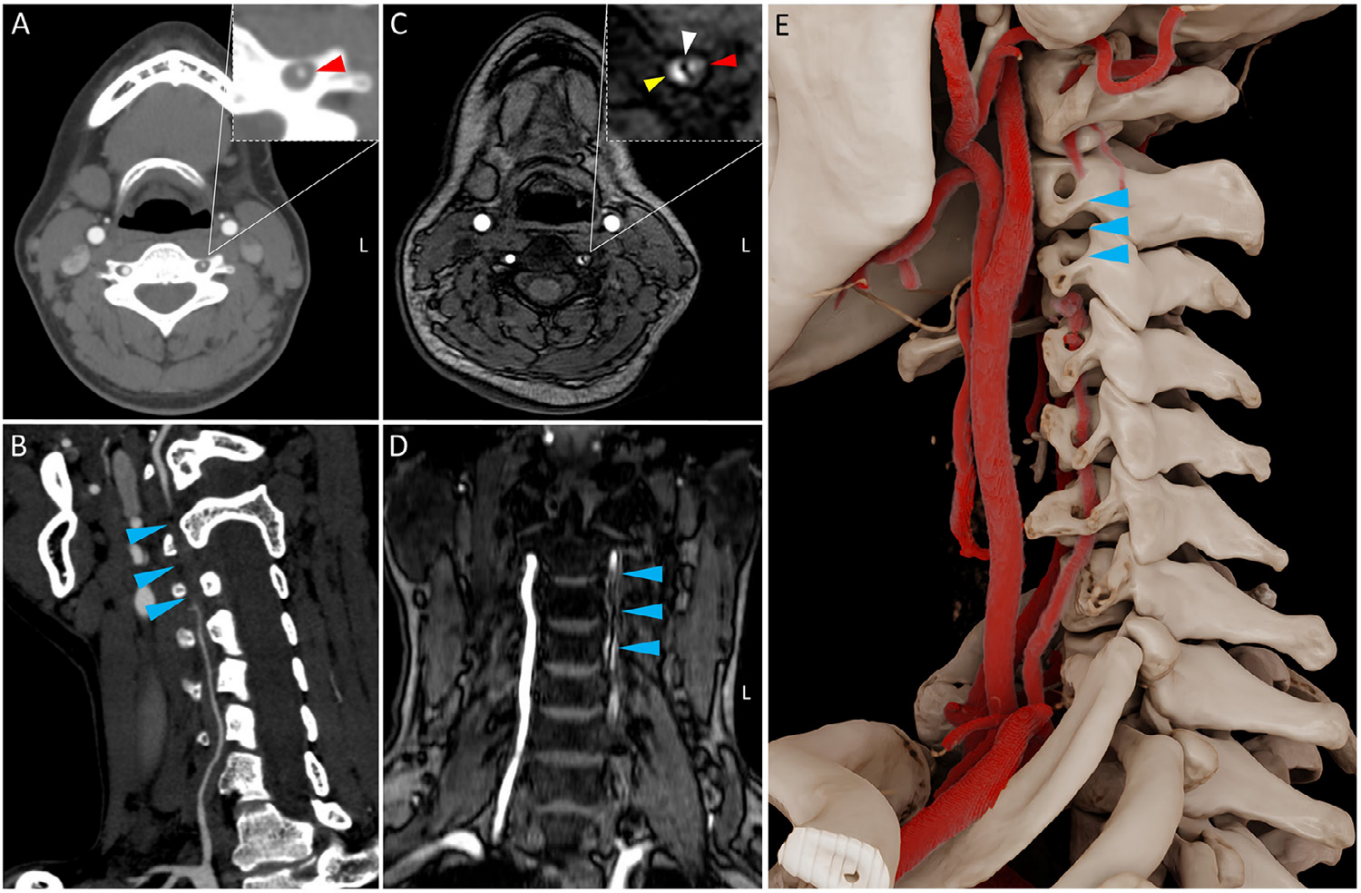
CTA and MRA findings of left vertebral artery dissection.
